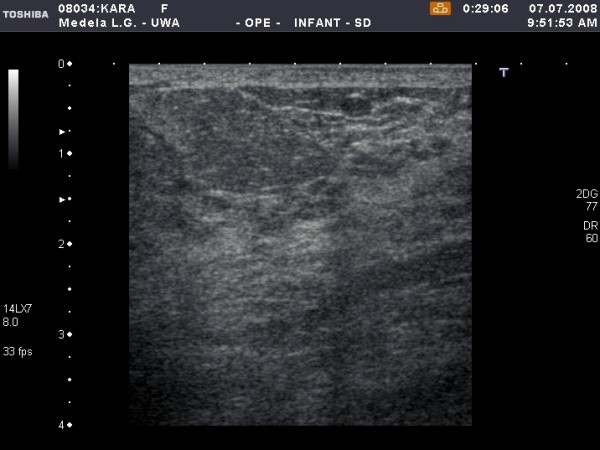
Image of the left lactating breast at position 12 o'clock after a breastfeed. The infant removed 58 g of milk. Ultrasonically there is a decrease in both the echogenicity and thickness of the glandular tissue compared to Figure 9.
